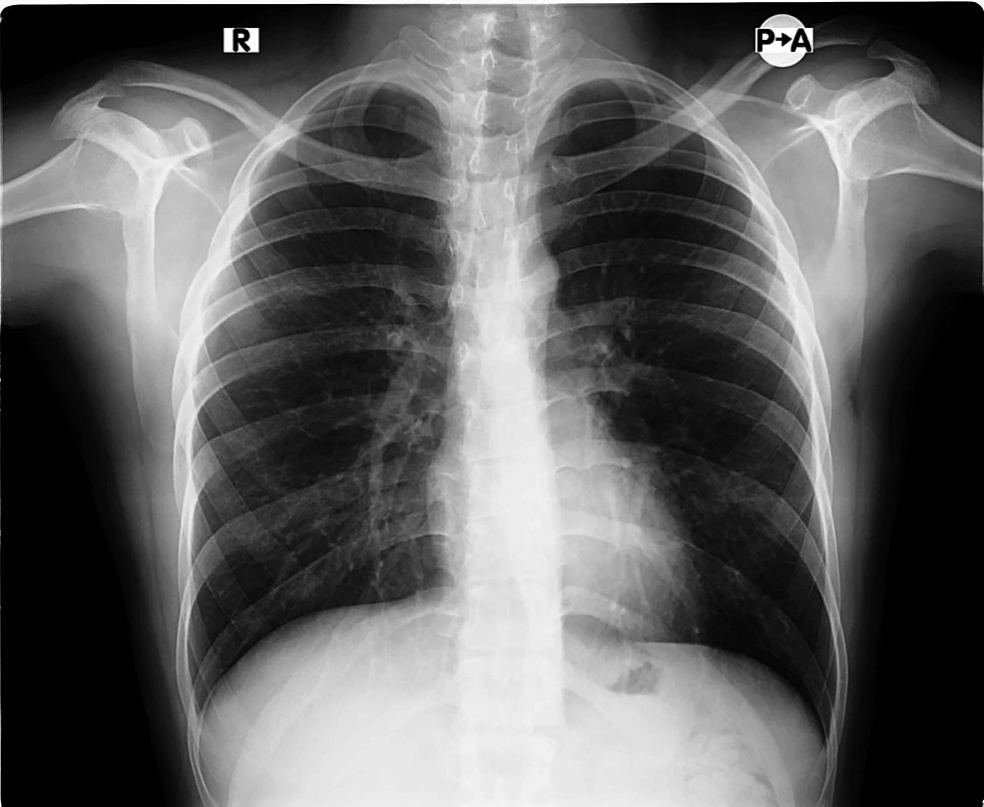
Chest X-ray showing a normal cardiothoracic ratio.
